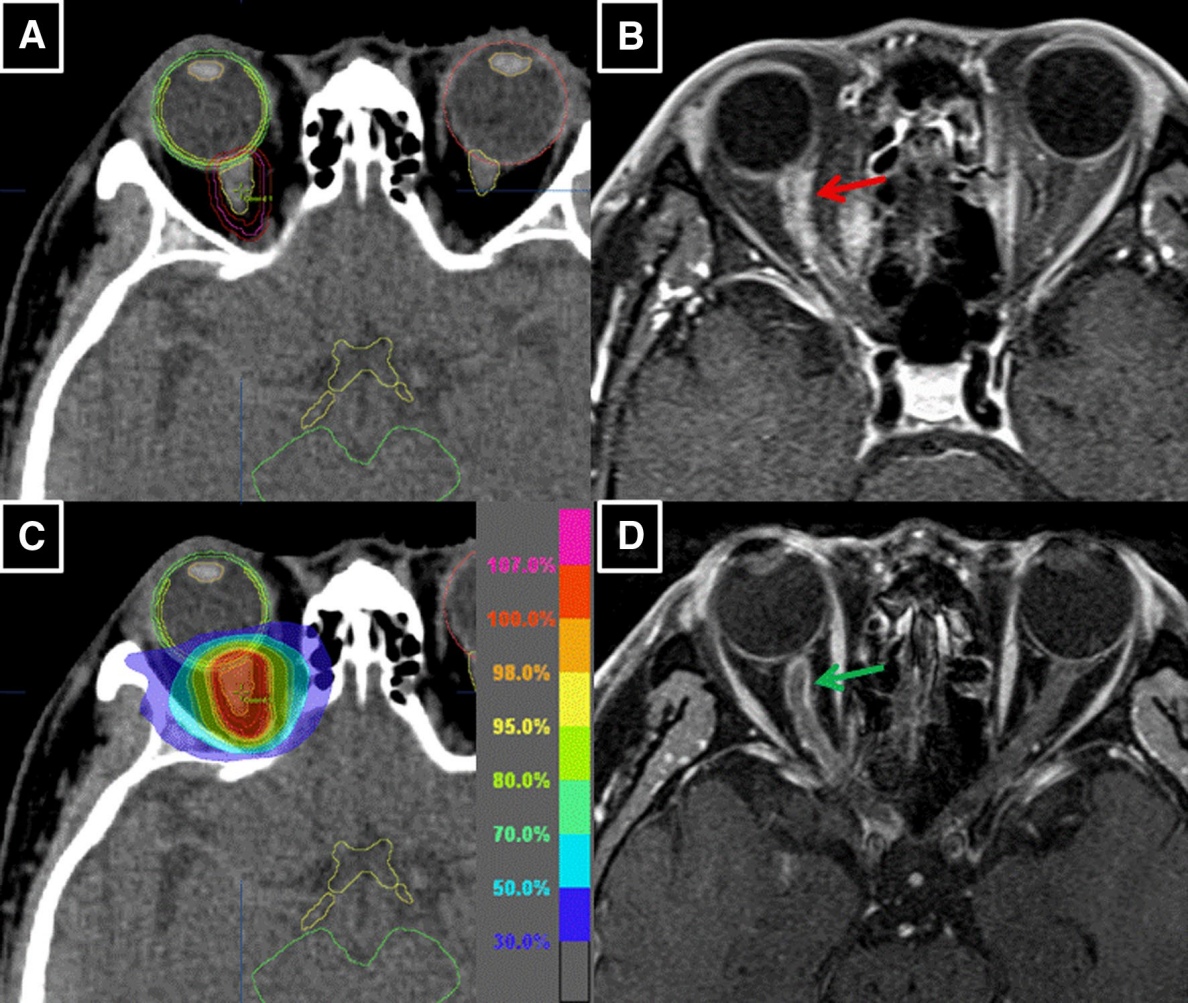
Patient #4 was a 61-year-old man. Pretreatment CT (a) and MRI showed a tubular intraorbital tumor (red arrow) of 8 × 3 mm located on the proximal portion of the right optic nerve. A “tram-track” sign was also detected (b). He underwent IMRT of 46 Gy in 23 fractions over 31 days in February 2015 (c). MRI taken in August 2015 revealed 53% reduction of the tumor volume (green arrow) (d)
